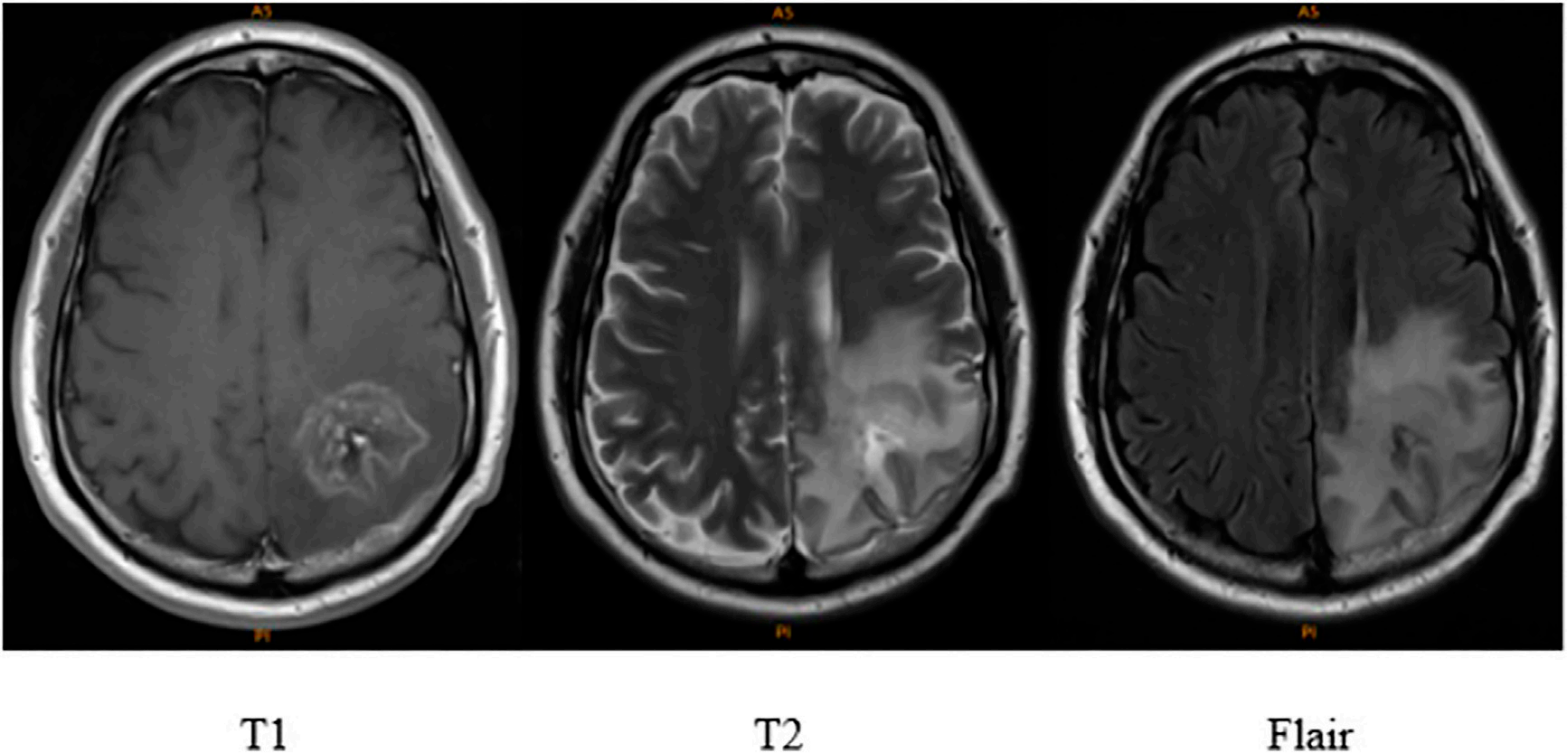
Comparison of T1, T2 and flair of brain tumors MRI (Clark et al., 2013; Scarpace et al., 2022).
